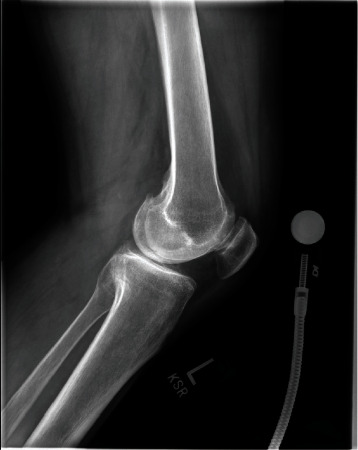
Preop lateral knee X-ray.
